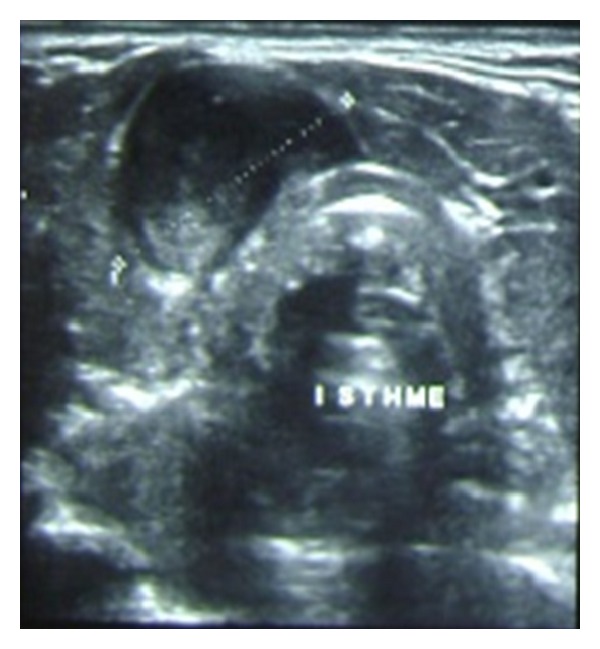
Neck ultrasonography that shows a heterogenous isthmic nodule.
